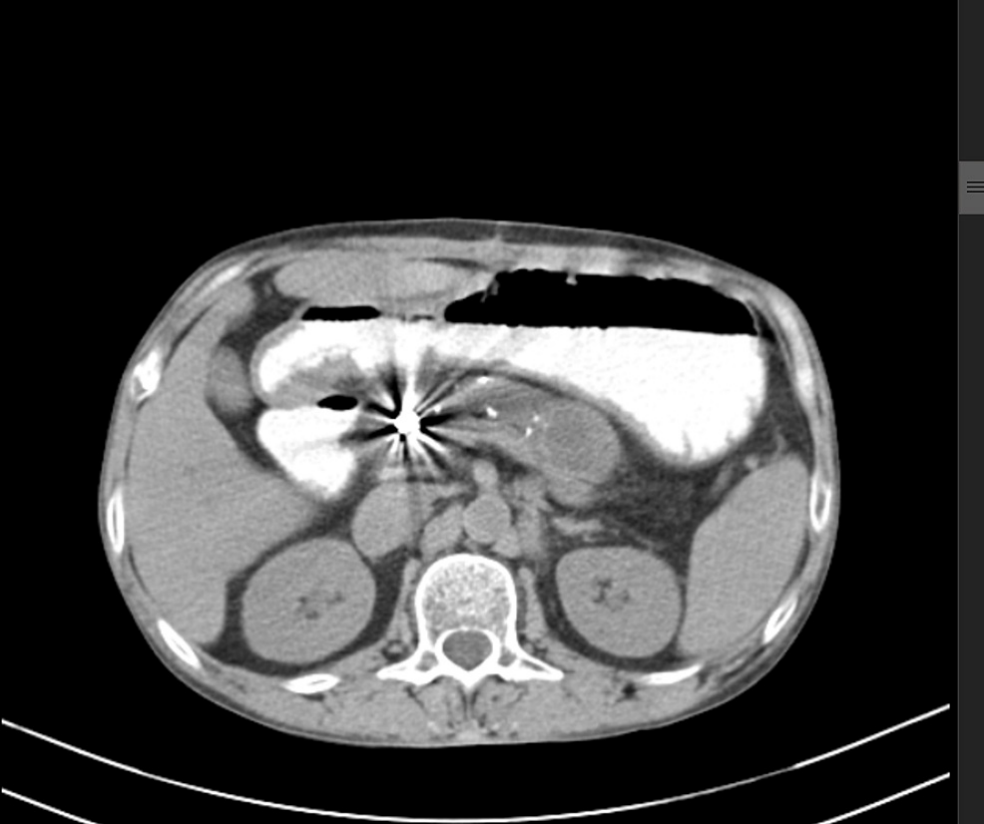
CECT abdomen showing chronic pancreatitis with calcification and pseudocyst involving the tail of pancreas. CECT, contrast-enhanced computed tomography of the abdomen
